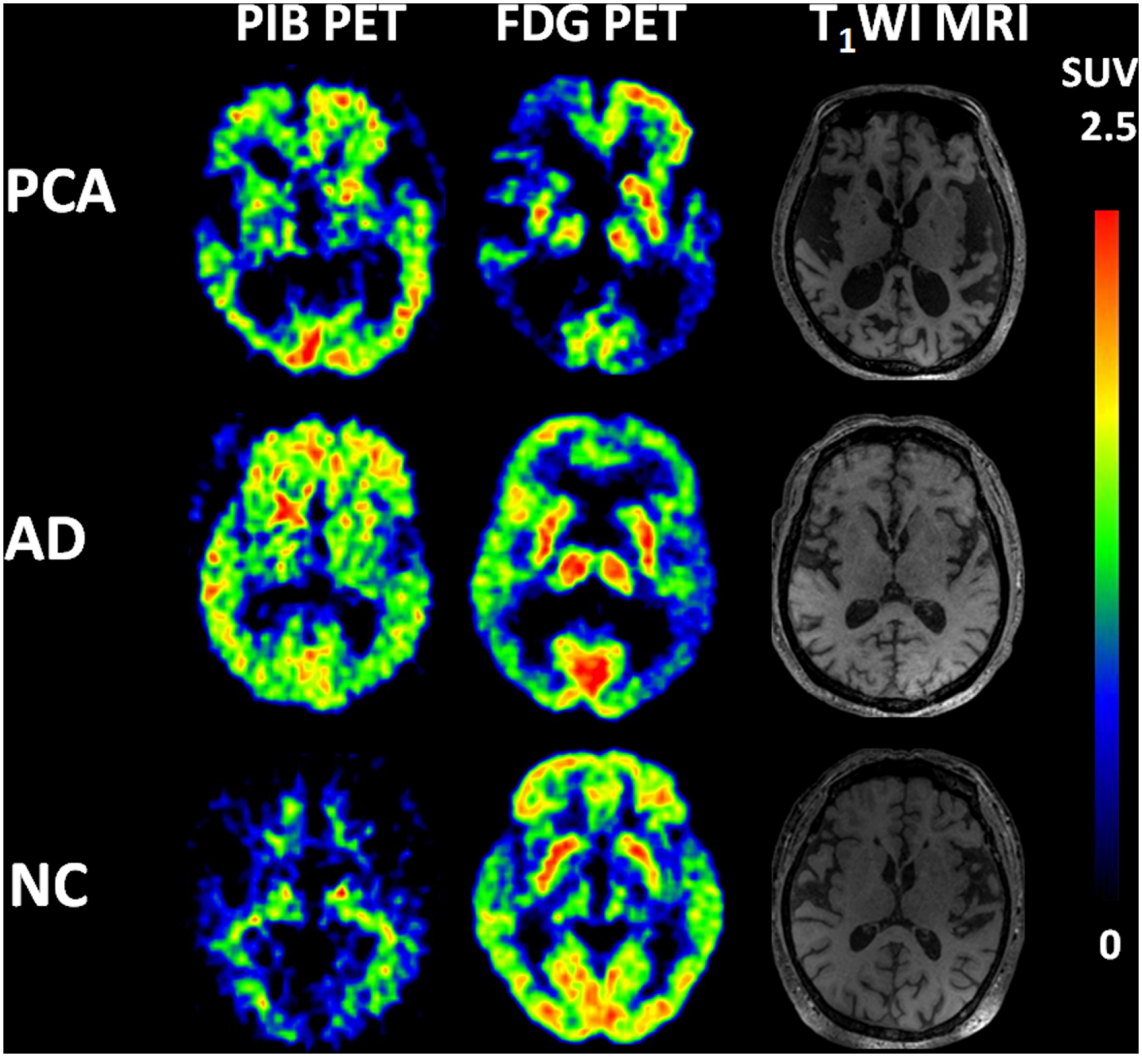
PiB (left), FDG (right) and MRI images in PCA and TAD patients. PiB and FDG images are quantified by SUVR with the displayed color scales. In both PCA and TAD patients, the clearance rate of radioactivity was slower symmetrically or asymmetrically in the cortex of frontal lobe, parietal lobe, lateral temperal lobe, praecuneus, posterior cingulate and occipital lobe.
